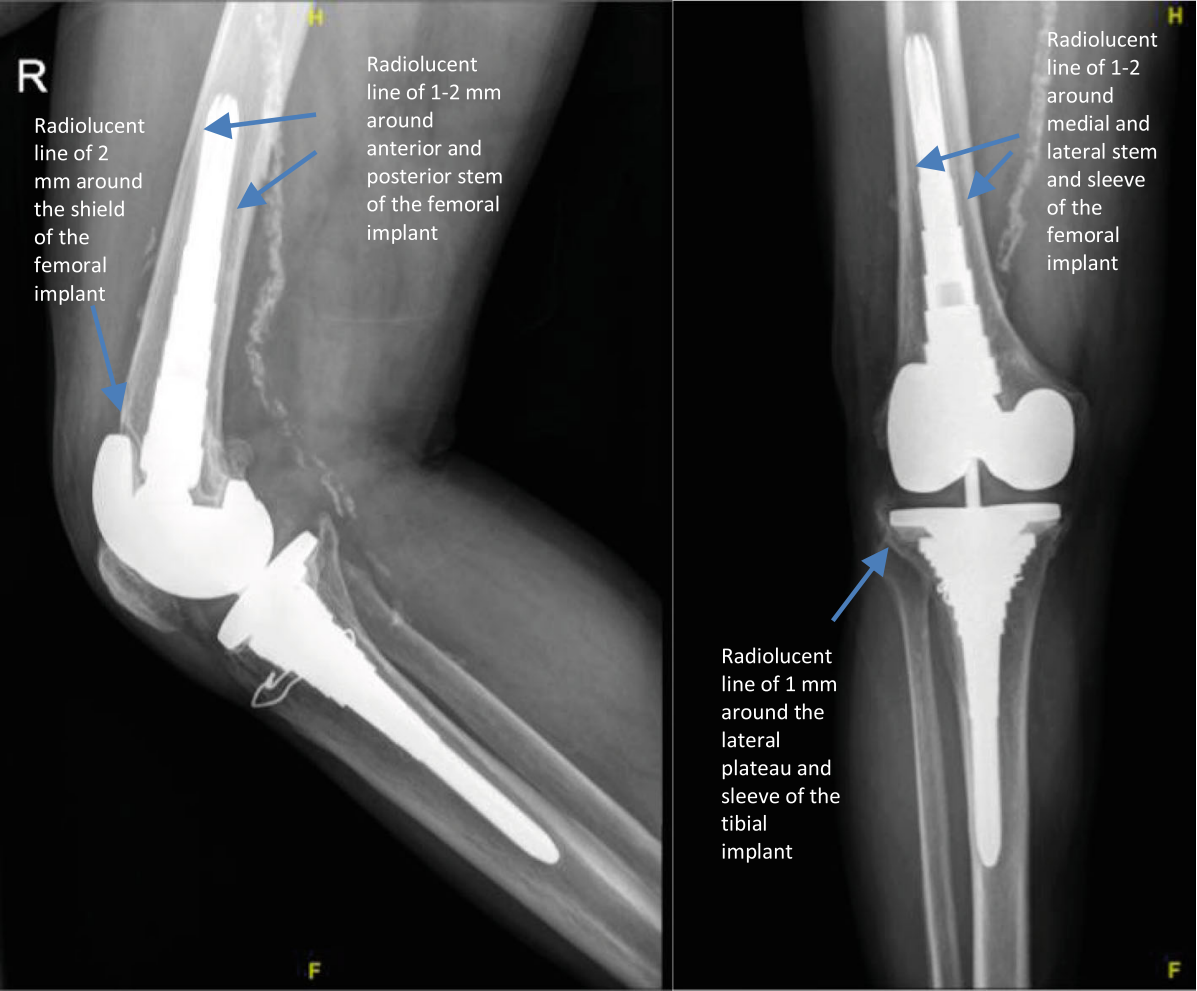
Antero-posterior (AP) and lateral view of the right knee of a 68-year old patient who underwent a second-stage revision total knee arthroplasty. A non-progressive radiolucent line of 1–2 mm around the stem, the anterior part of the femoral sleeve and the tibial plate are seen in radiographs taken 7 years postoperatively. Clinically, the patient had no signs of loosening
